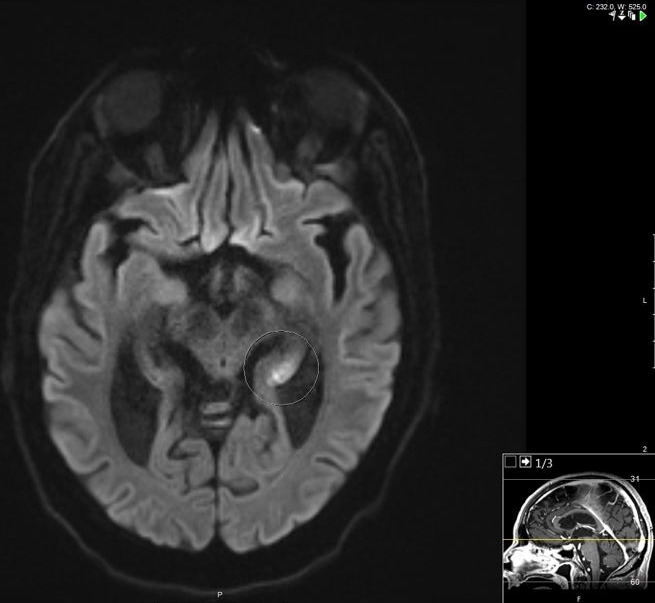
A magnetic resonance imaging (MRI) brain showing an abnormal hyperintense signal involving the tail of the left hippocampus on diffusion weighted image (DWI) sequence
